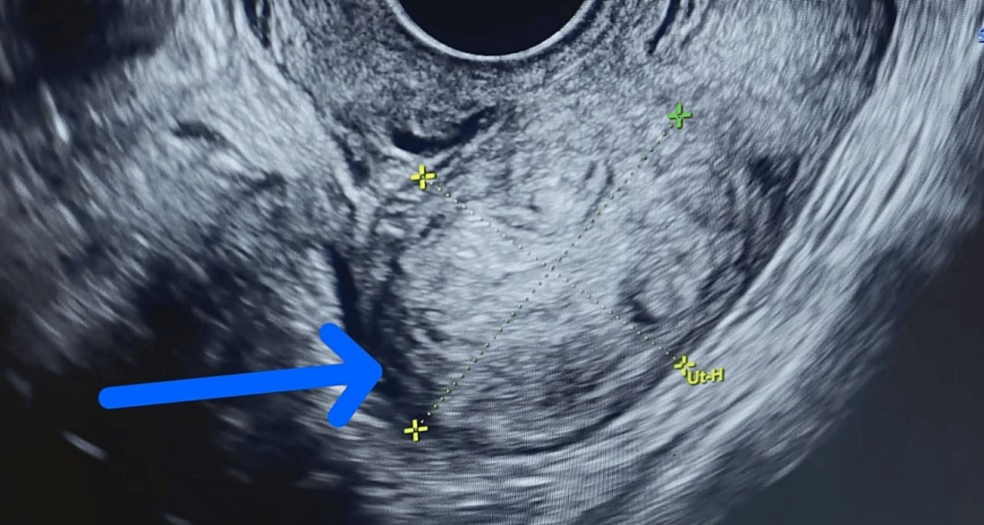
Ultrasonographic image of the suspected uterine fibroid mass
